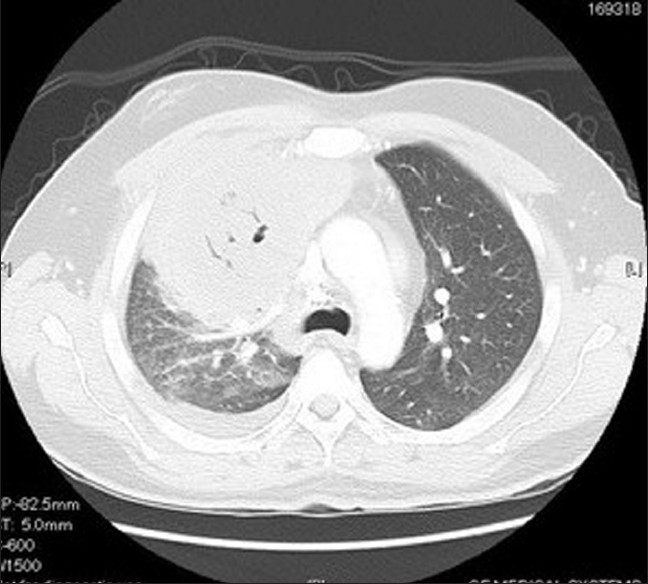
Computed tomography of the chest showing large mass with central hypodensity involving right upper lobe
